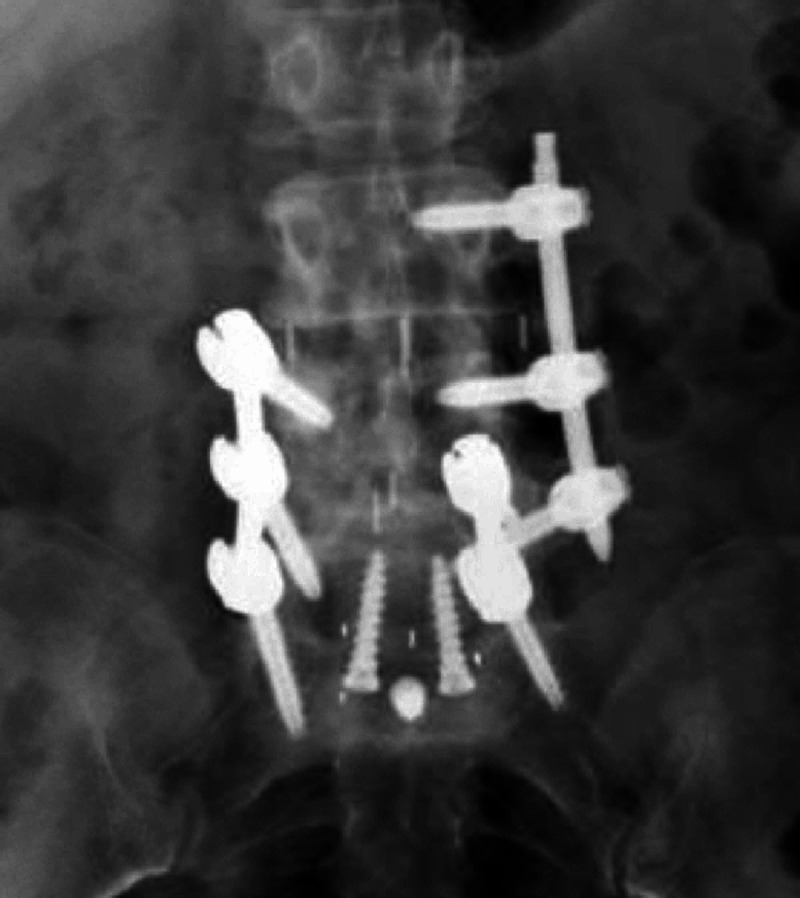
Postoperative anterior-posterior X-ray demonstrating right-sided traditional pedicle posterior instrumentation from L4-S1 on the right side. On the left side is an additional posterior construct at L5-S1 with a cortical bone trajectory screw at the same level as a traditional pedicle crew in the left L5 pedicle
